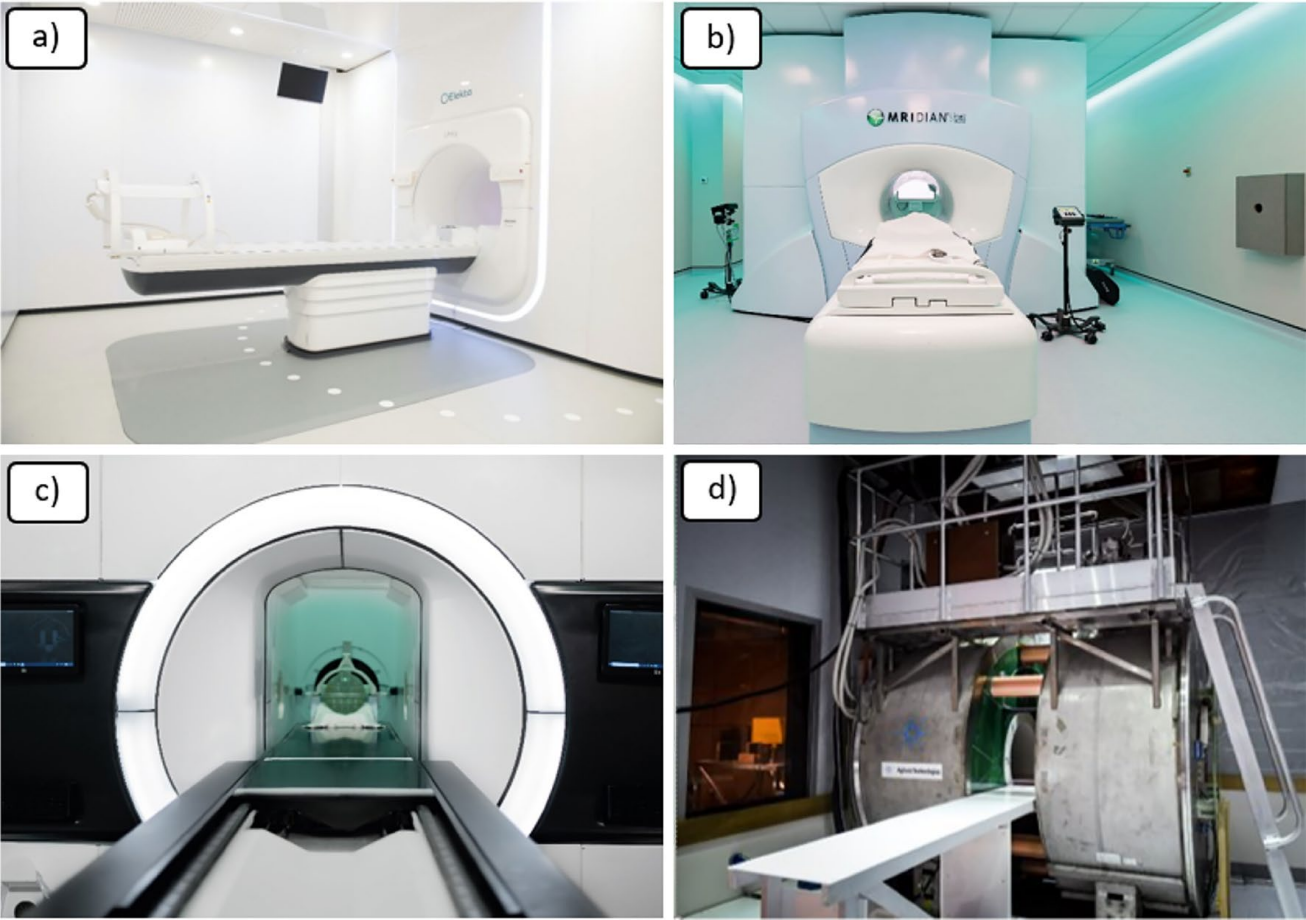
Current MRI-linac systems a Elekta Unity, b ViewRay MRIdian, c MagnetTx Aurora and d Australian MRI-linac
